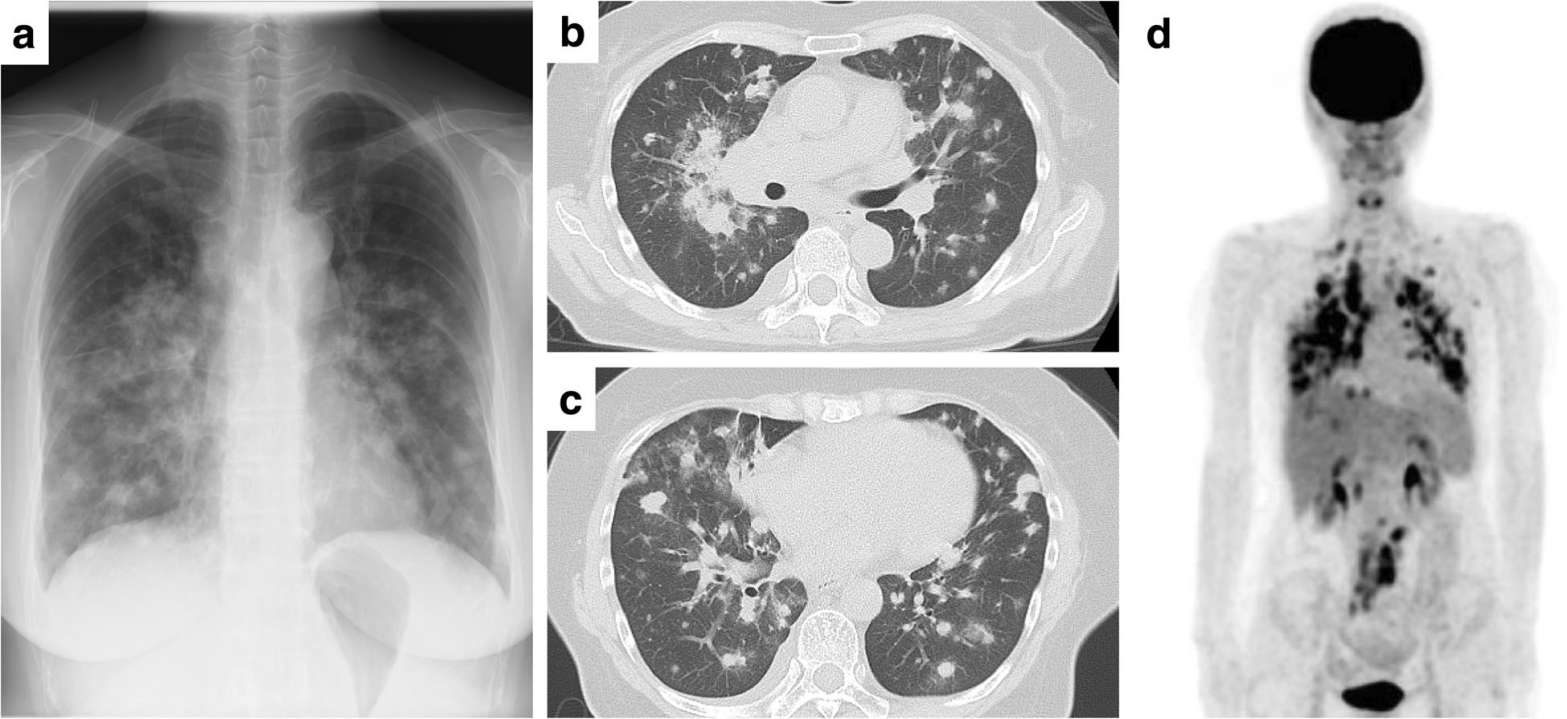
Radiographic features of T-cell lymphoma. a-c Chest X-ray and a computed tomography scan showed multifocal pulmonary consolidations and nodules. d 18F-fluorodeoxyglucose positron emission tomography (FDG-PET) scan showed the accumulation of FDG in the bilateral lung nodules and the lymph nodes
